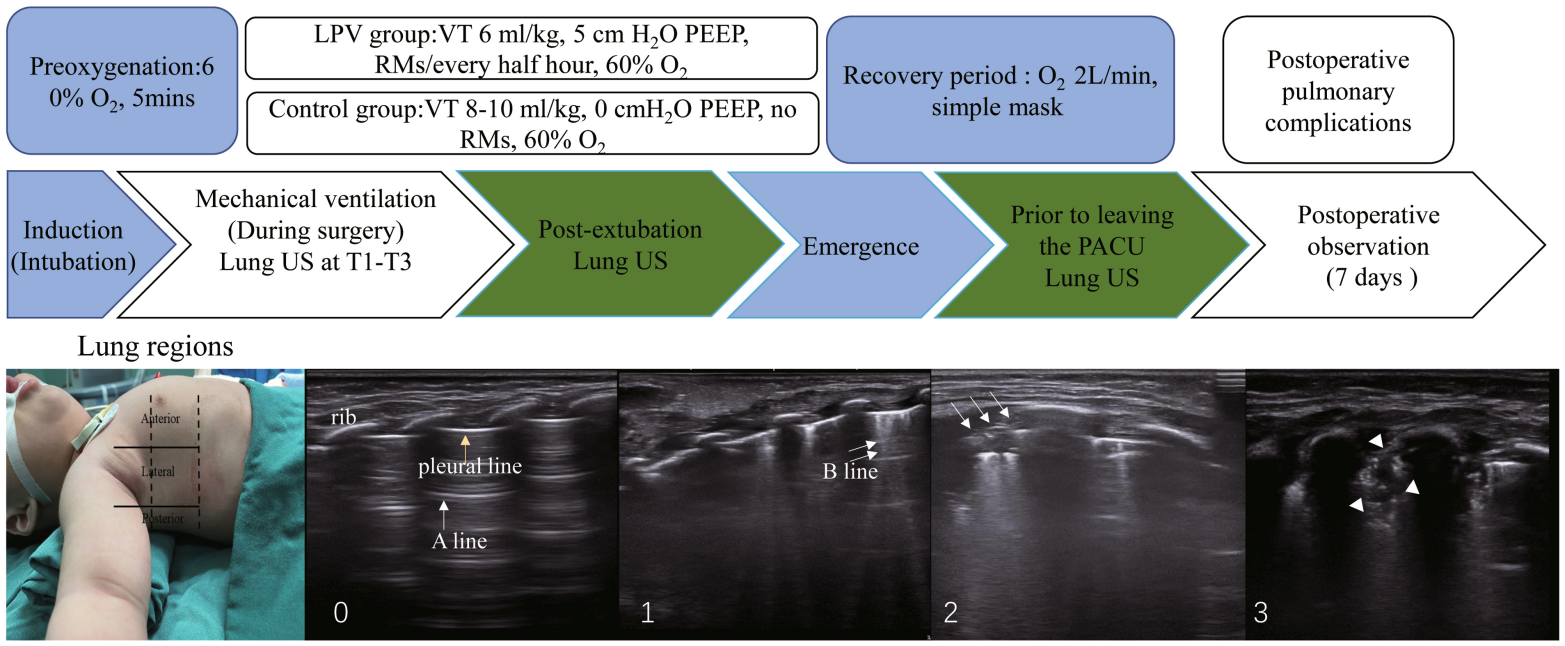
The study protocol and ultrasound lung examination region is shown. From left to right are scores: 0, 0 to 2 B lines; 1, at least three B lines or one or multiple small subpleural consolidations separated by a normal pleural line; 2, multiple coalescent B lines or multiple small subpleural consolidations separated by a thickened or irregular pleural line; 3, consolidation or small subpleural consolidation of more than 1 cm x 2 cm. One yellow arrow, pleural line; one white arrow, A line; two white arrows, B line; three white arrows, subpleural consolidations; white arrowheads, consolidation. Significant atelectasis is determined if any region had a consolidation score of 2. RMs, recruitment maneuvers, PEEP, positive end-expiratory pressure. T1, 5 min post-intubation, T2, 5 min post-pneumoperitoneum, T3, at the end of surgery.
